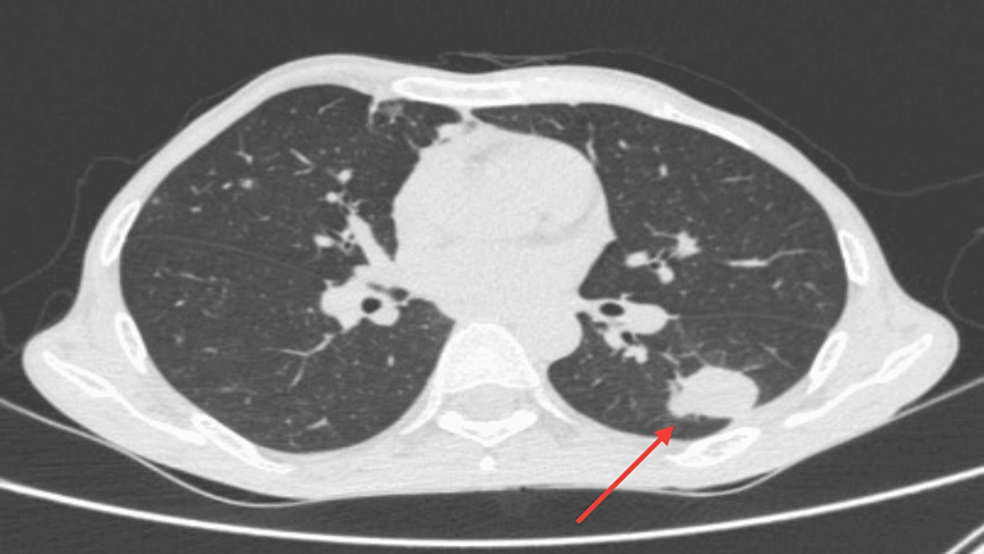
Single ill-defined pulmonary nodule (seen with red arrow) with an irregular spiculated margin showing subtle peripheral post-contrast enhancement with subpleural thickening seen in adjacent pleura in the left lower lobe in the superior segment as seen in this axial CT thorax section.
